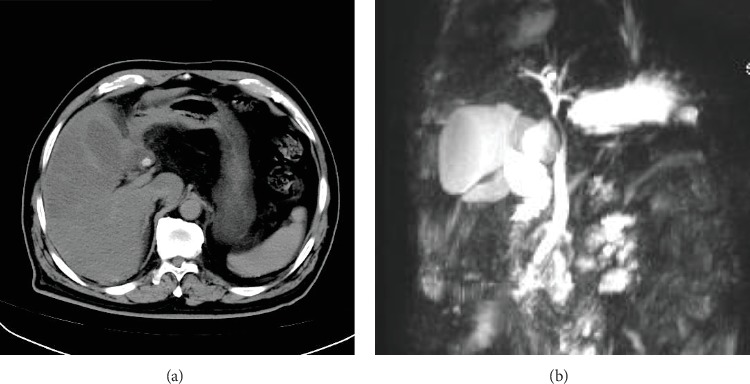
Preoperative imaging of Case 2. (a) A computerized tomography (CT) scan shows gallbladder enlargement and dilation of the intrahepatic bile duct. (b) Magnetic resonance cholangiopancreatography (MRCP) imaging shows external compression of the common bile duct by a cystic duct stone.
